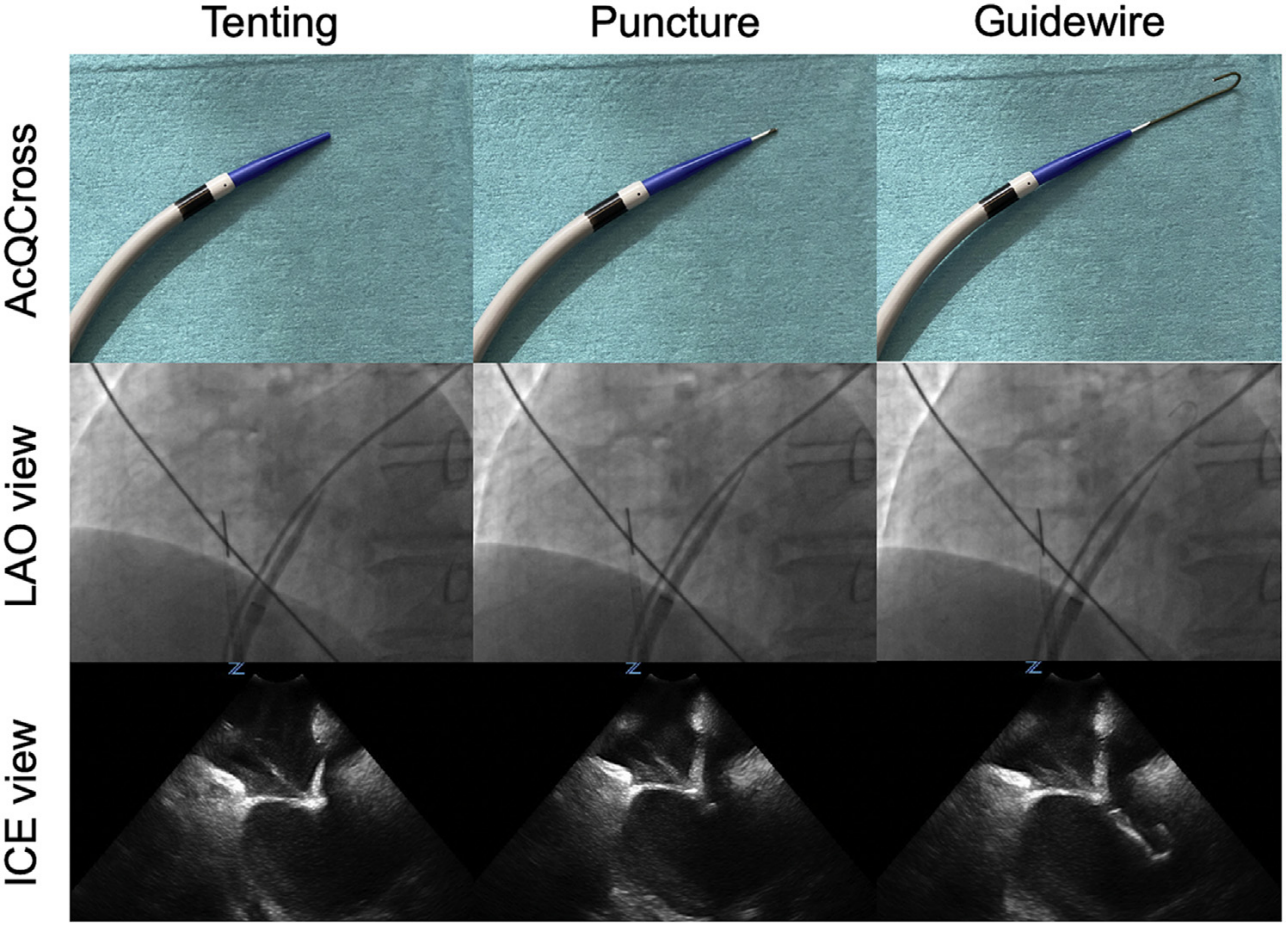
Imaging of the AcQCross dilator/needle system (Acutus Medical, Carlsbad, CA) during the different steps of a transseptal puncture. After visualization of tenting of the fossa ovalis during intracardiac ultrasound imaging, the needle is advanced and the retained 0.032” guidewire is advanced to the left superior pulmonary vein. ICE = intracardiac echocardiography; LAO = left anterior oblique.
